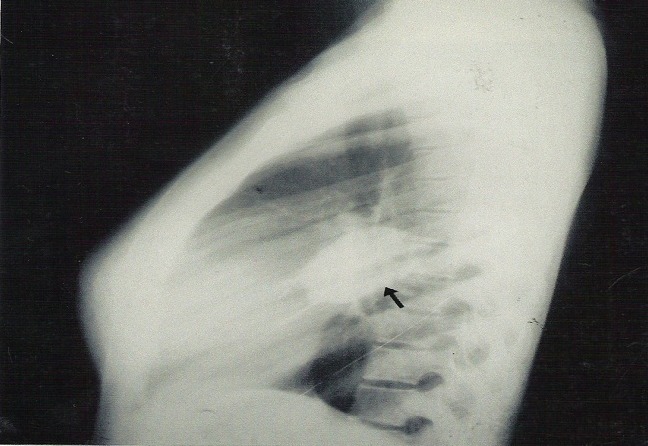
Lateral chest radiograph showing a well defined oval shaped homogenous opacity in the fissure
